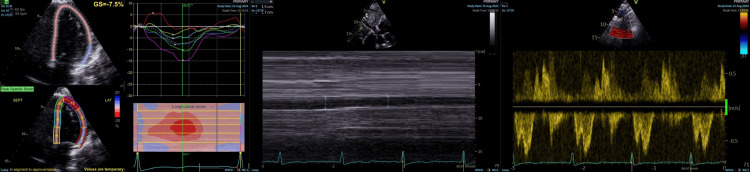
Speckle-tracking echocardiography and Doppler assessment of LV function Severely reduced LV systolic function (EF 25%–30%) with global strain of –8% and regional variability. LVOT VTI 8.5 cm; LV size normal, no pericardial effusion. LV: left ventricular; EF: ejection fraction; LVOT: LV outflow tract; VTI: velocity time integral
